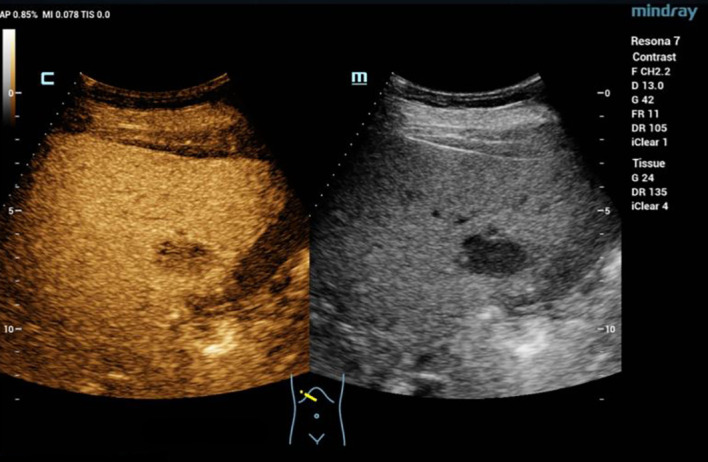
Abdominal ultrasonography revealed a hypoechoic mass in the right anterior and inferior segment of the liver, about 2.9 × 1.8 cm in size, with clear boundaries and relatively regular shape.
